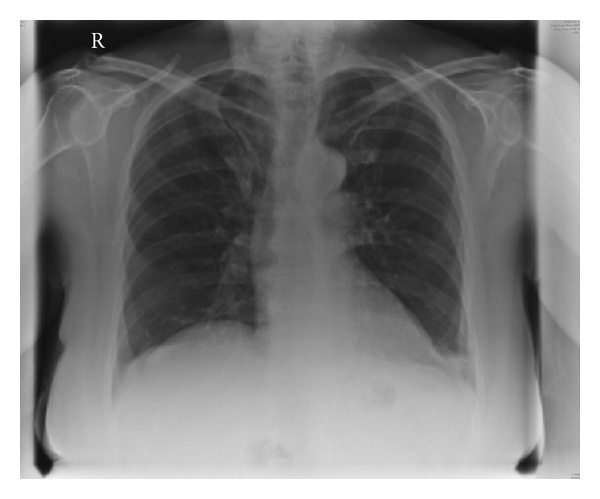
Anteroposterior chest radiograph demonstrating deviation of the trachea around right mediastinal mass.
